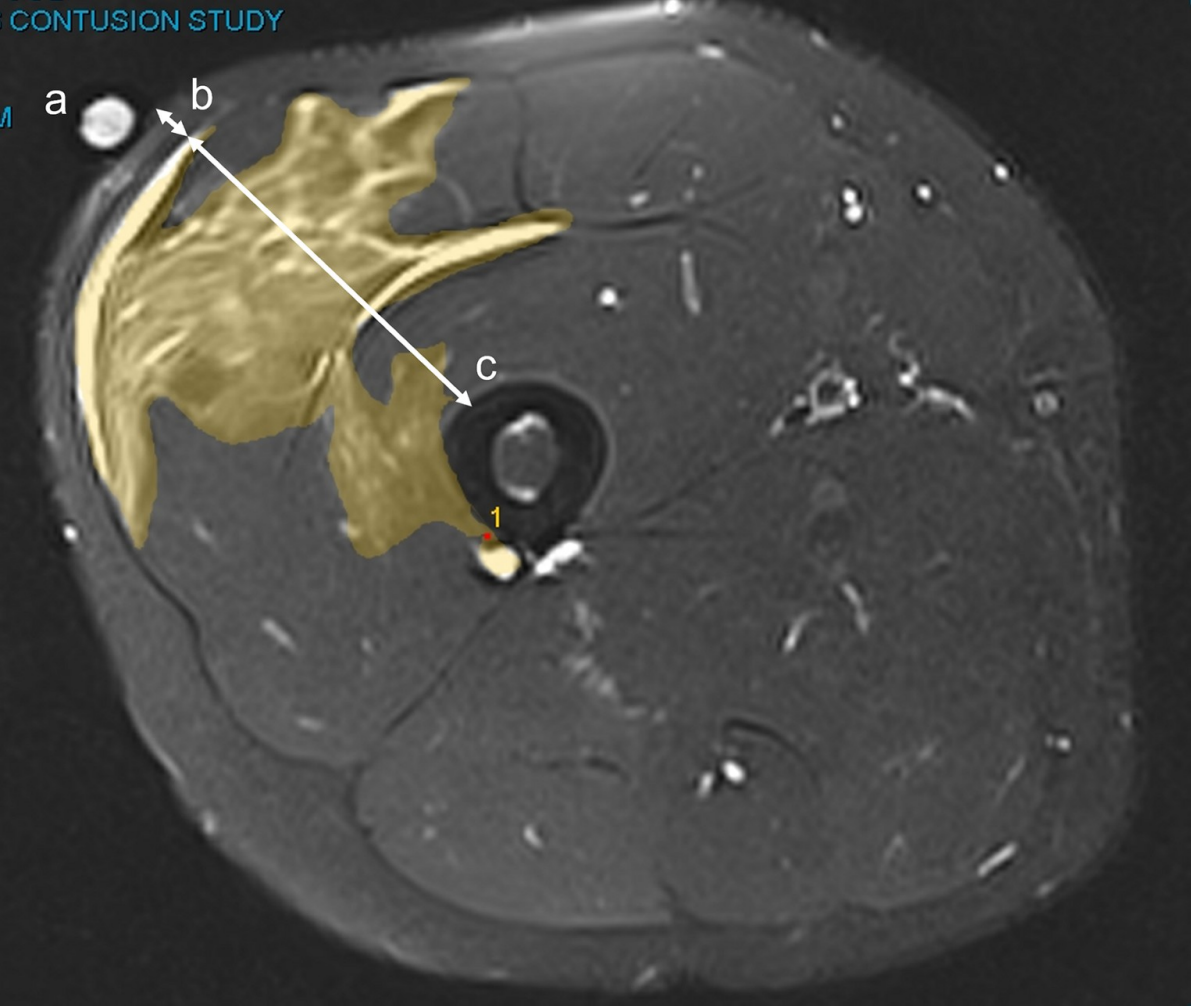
MRI image, at 24 h post-impact, of oedema in the quadriceps after impact with 7.2 kg. Oedema is identified as regions of high intensity and area measured (highlighted area) so that oedema volume could be quantified. Additionally, fat (b) and muscle thickness (c) were measured at the site of impact. (a) denotes the vitamin E capsule marker placed on the centre of impact. Axial T2-weighted TSE SPAIR FS (TR 4160 ms; TE 61 ms; FOV 230 × 230 mm; slice thickness 4 mm; interslice gap 0 mm) was used for imaging.
